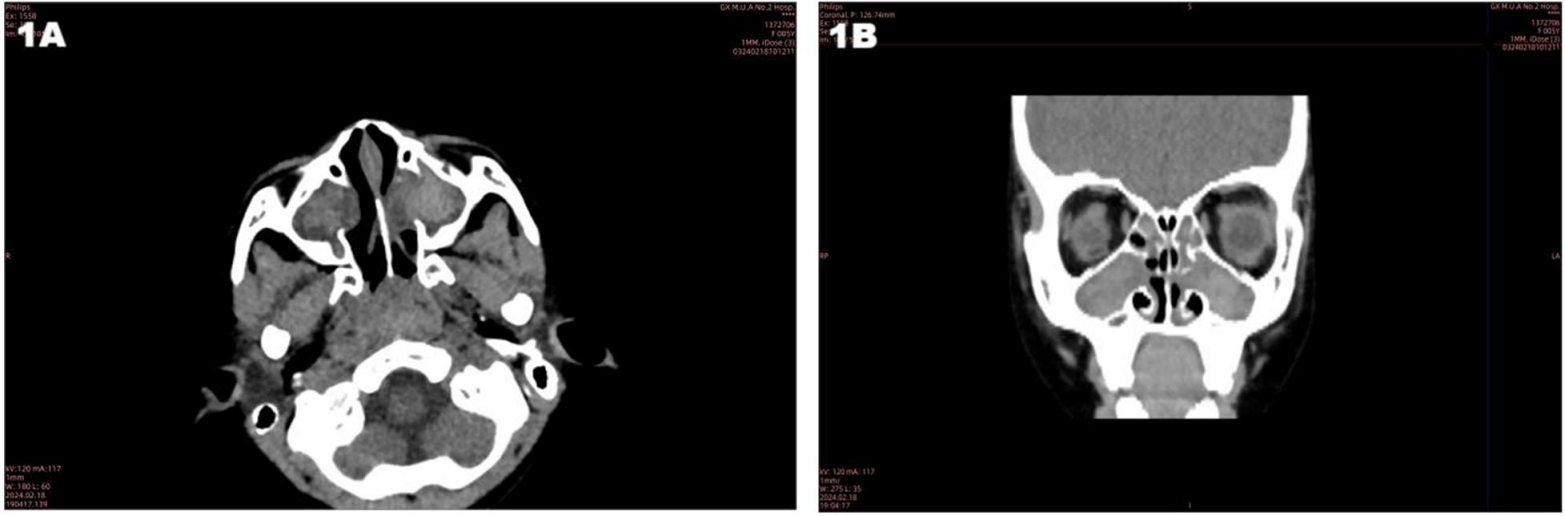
CT of nasal sinuses: sinusitis. (A) Coronal view of the paranasal sinuses. The image shows obvious thickening of the mucous membrane in the maxillary sinuses and ethmoid sinuse, with partial narrowing of the sinus ostia-meatal complex. (B) Axial view of the paranasal sinuses. It further confirms the presence of sinusitis: the mucous membrane of the frontal sinuses and sphenoid sinuses is slightly thickened, and there is no obvious effusion in the sinus cavity or destruction of the sinus bony structure.
